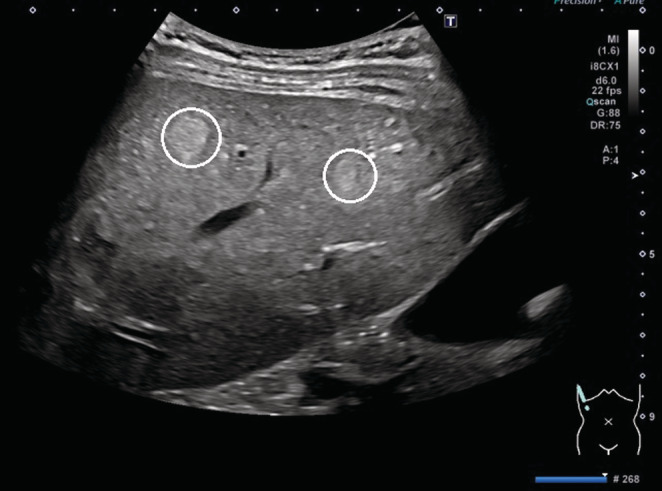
Grayscale liver ultrasonography showing nodular lesions (white circles) at 14 weeks of gestation.
